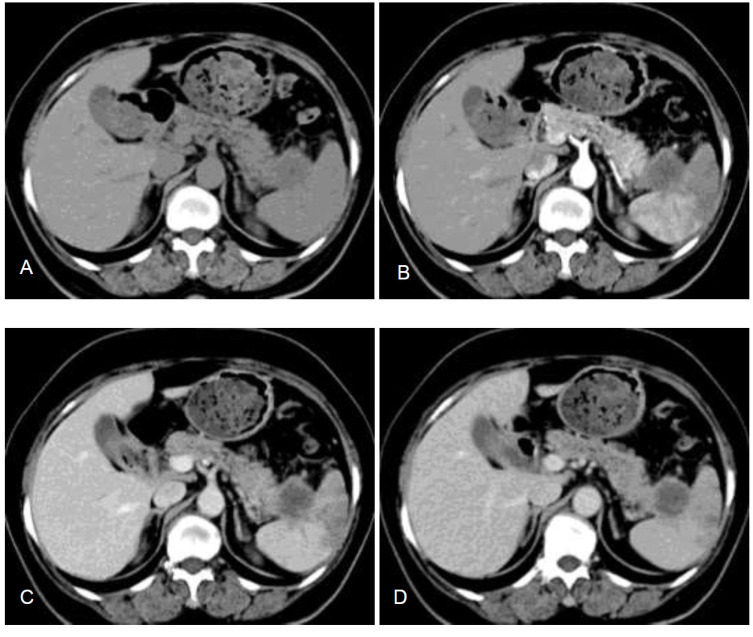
CT Imaging of Pancreatic Mucoepidermoid Carcinoma. A: CT scan demonstrates a round, hypodense mass in the tail of the pancreas. B-D: Post-contrast CT images during the arterial, portal venous, and delayed phases demonstrate peripheral ring-like delayed enhancement over time. A wedge-shaped hypodense area pointing toward the splenic hilum is seen within the spleen, suggestive of splenic infarction.
